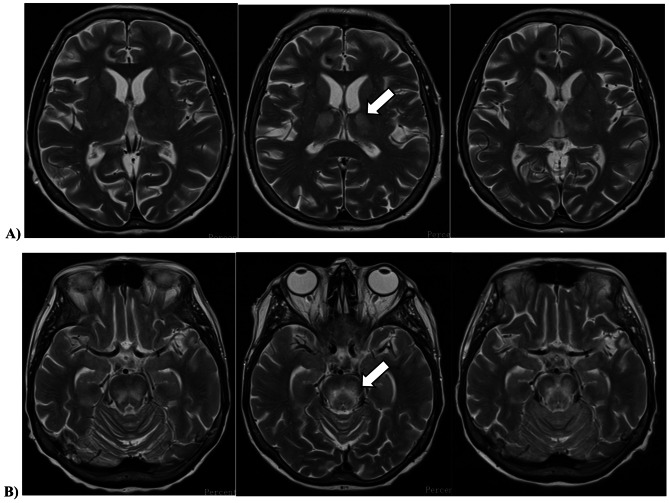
Cranial magnetic resonance imaging of the patient, showing focal progression with time. T2-weighted turbo spin echo sequences, acquired in the transverse planes of A) the thalamus and B) the midbrain
